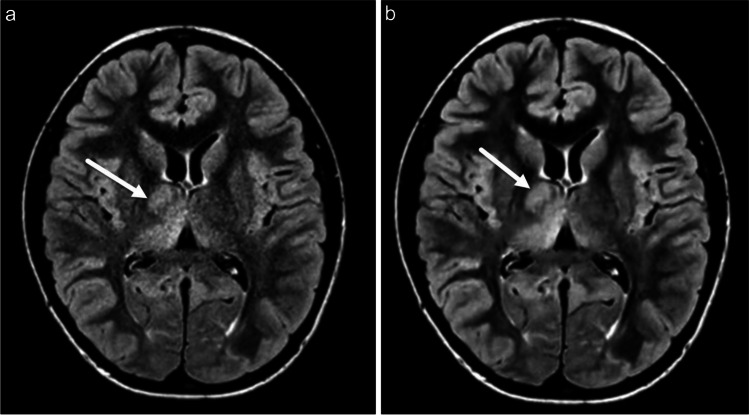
a-b Axial FLAIR CS=1.6 brain MR in a 14-year-old female presenting with new onset of headaches and dizziness without (a) and with (b) AI image reconstruction demonstrates global reduction in image noise and ill-defined signal abnormality in the right thalamus (arrows) suspicious for infiltrative neoplasm, reproduced on AI reconstruction
